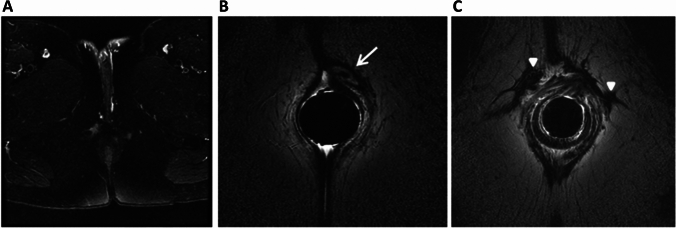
Pelvic Phased array versus endoluminal coils on postoperative MRI after incision and drainage of perianal abscess including seton drain placement. Axial T2-weighted (T2W) with fat suppression (A) and without (B, C). A On the external phased array image no evident lesions are seen; conversion to endoanal coil (1.5T) demonstrates a sub-sphincteric fistula with drain in situ (arrow), B including the patent ostia of two fistula tracts. C Arrowheads point at fibrotic remnants of dried up fistula tracts
